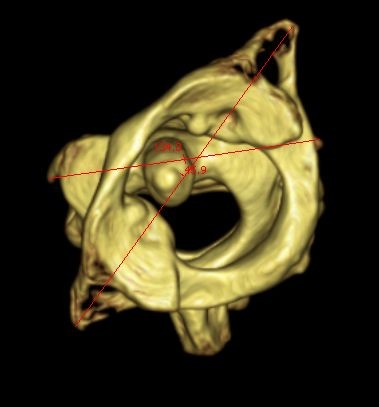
Atlantoaxial computed tomography scan. Atlantoaxial rotation of 46° to the left.
